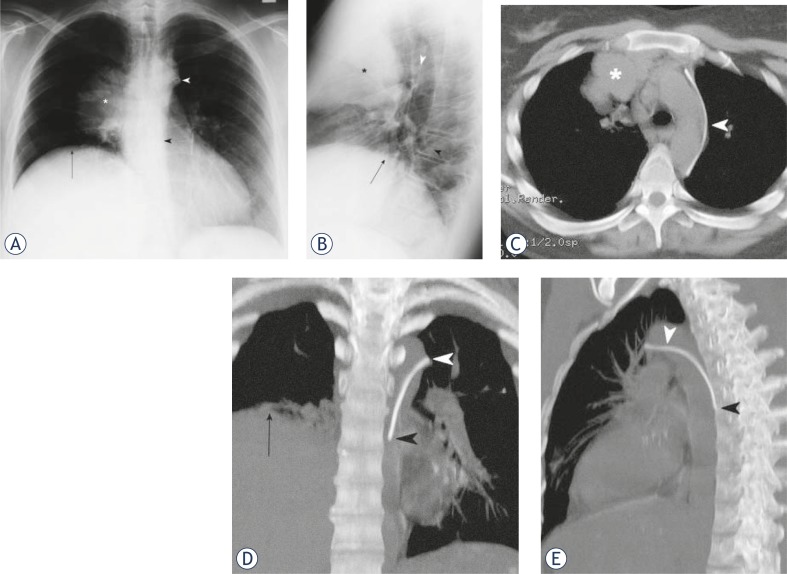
Posteroanterior (A) and lateral (B) chest X-ray. Axial (C), coronal (D) and sagittal (E) maximum intensity projection (MIP) of non-enhanced thoracic CT scan. Catheter inserted through the left subclavian vein arcs laterally in the left superior intercostal vein (white arrowhead) and then descends medially in the posterior mediastinum through the accessory hemiazygos vein (black arrowhead). Bulky mediastinal mass (asterisk) causing right phrenic nerve palsy and elevation of the right hemidiaphragm (arrow).
